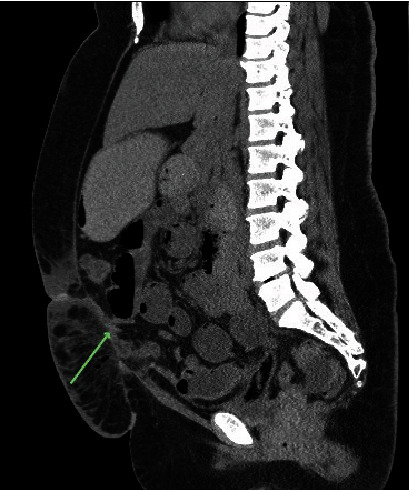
CT abdomen and pelvis showing a markedly distended stomach and proximal jejunum with a transition zone within the rectus abdominis musculature.
